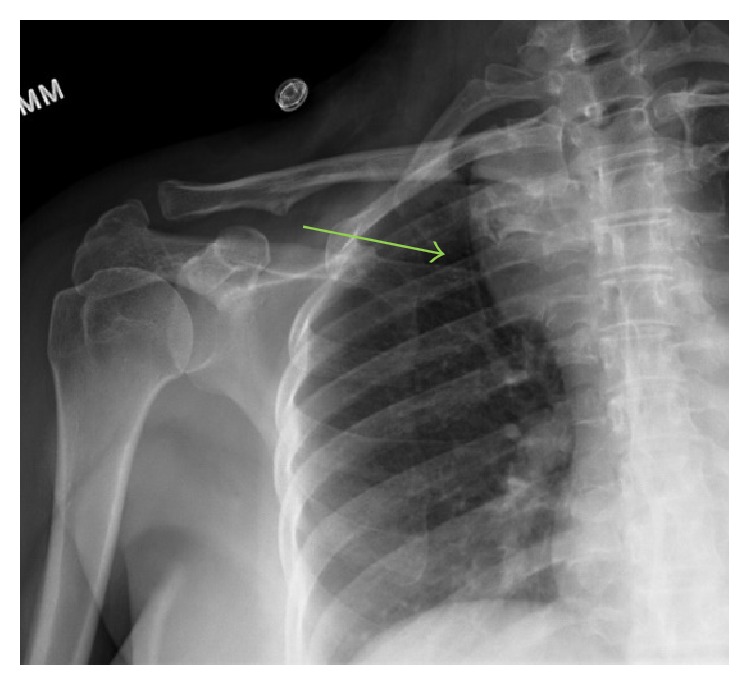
Frontal radiograph of the right shoulder shows a superior mediastinal mass (green arrow) within the right hemithorax.
